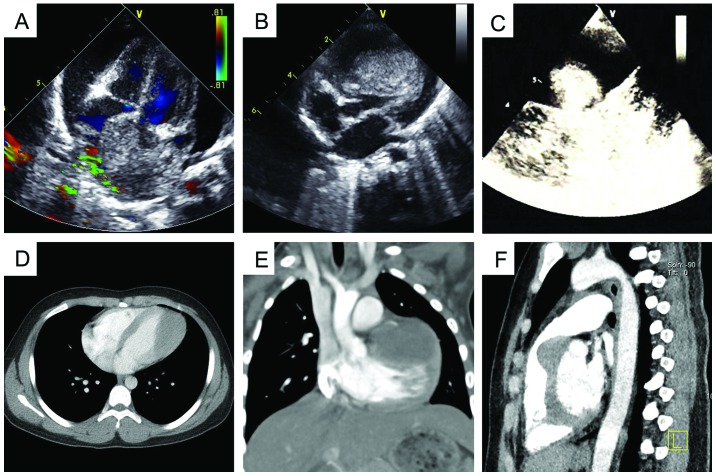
Echocardiography and computed tomography angiography prior to surgery. (A) Tumor in the atrial septum with moderate pericardial effusion. (B) Right ventricular tumor with outflow tract obstruction. (C) Intrapericardial mass. (D) Left ventricle tumor. (E) Left ventricle tumor with left coronary artery involvement. (F) Right ventricular tumor.
